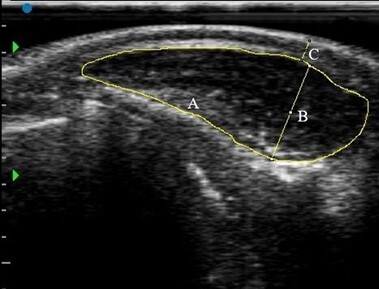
The real-time ultrasound image and its interpretation in a buffalo calf (borders A and depth B of longissimus muscle, and subcutaneous fat thickness (SFT) labeled C).
